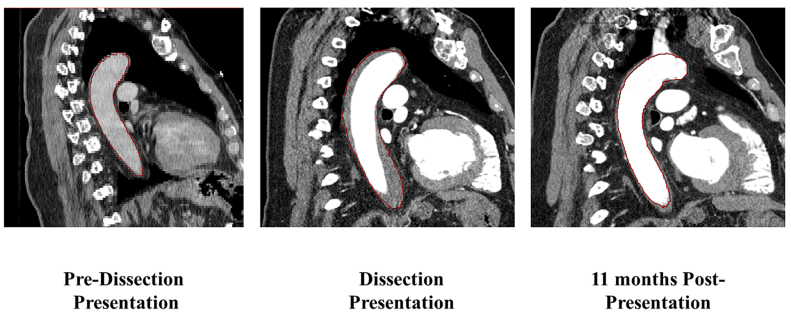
Computed tomography (CT) image data evolution. Sagittal view of patient CT image data for three clinically relevant timepoints. *Red* outline indicates boundaries of thoracic aorta segmentation.
